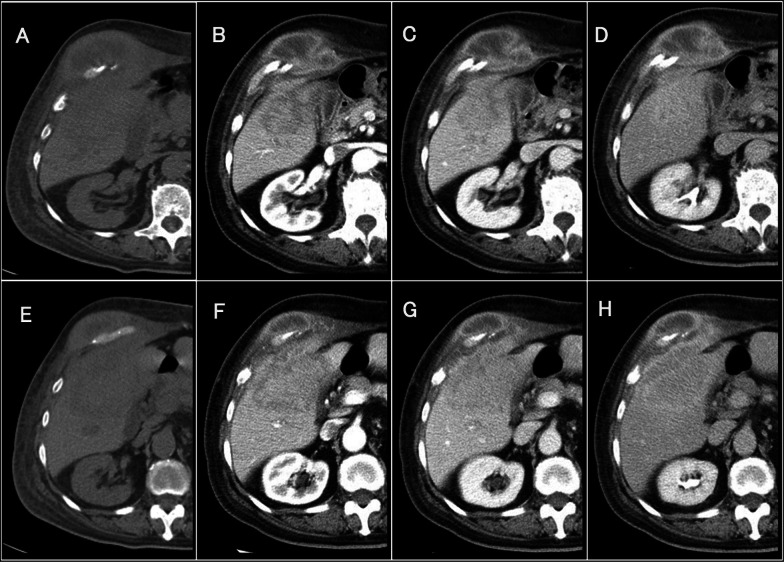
Abdominal computed tomography images before admission (A, E plain, B, F arterial phase, C, G portal phase, D, H equilibrium phase; A–D cranial site of the mass, and E–H caudal site of the mass)
